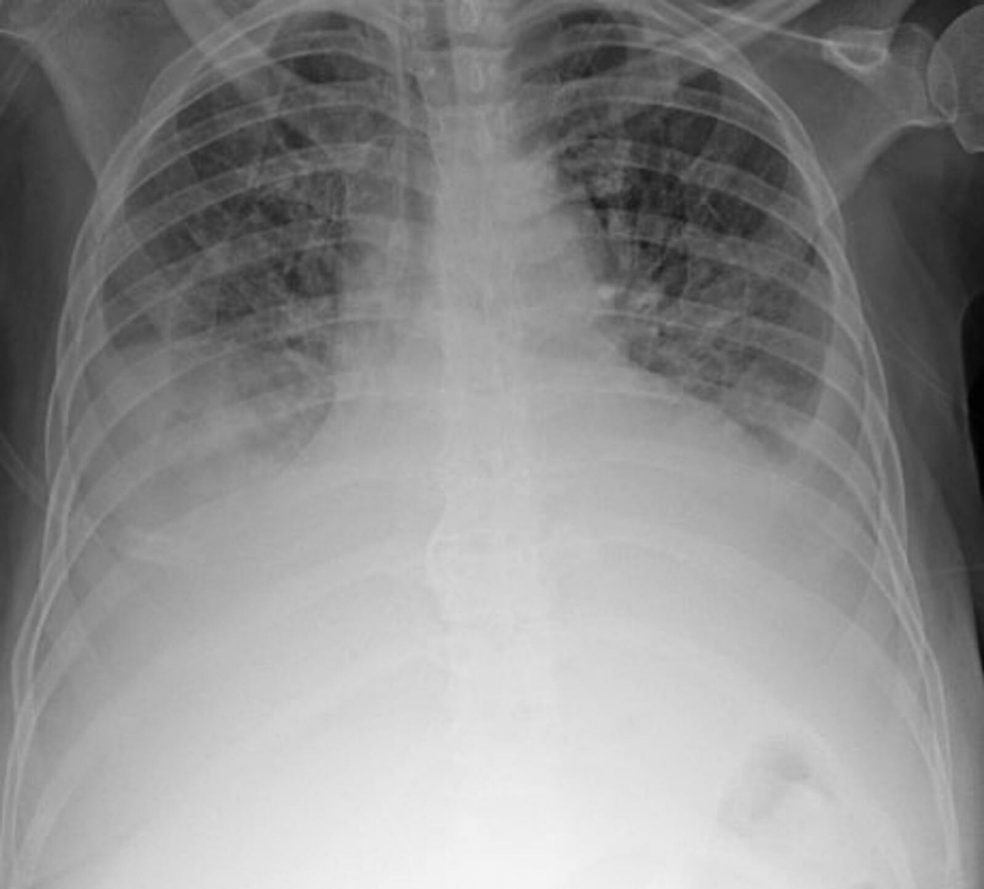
Initial chest X-ray showing bilateral pleural effusions with a significant right pleural effusion, cardiomegaly, and bilateral opacities.
